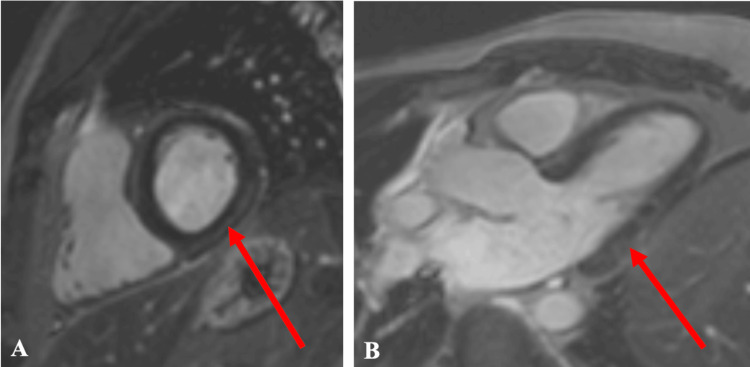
Cardiac magnetic resonance imaging (A-B). Late gadolinium-enhanced imaging reveals subepicardial fibrosis (indicated by red arrows) confined to the basal and mid segments of the inferior and posterior walls of the left ventricle.
